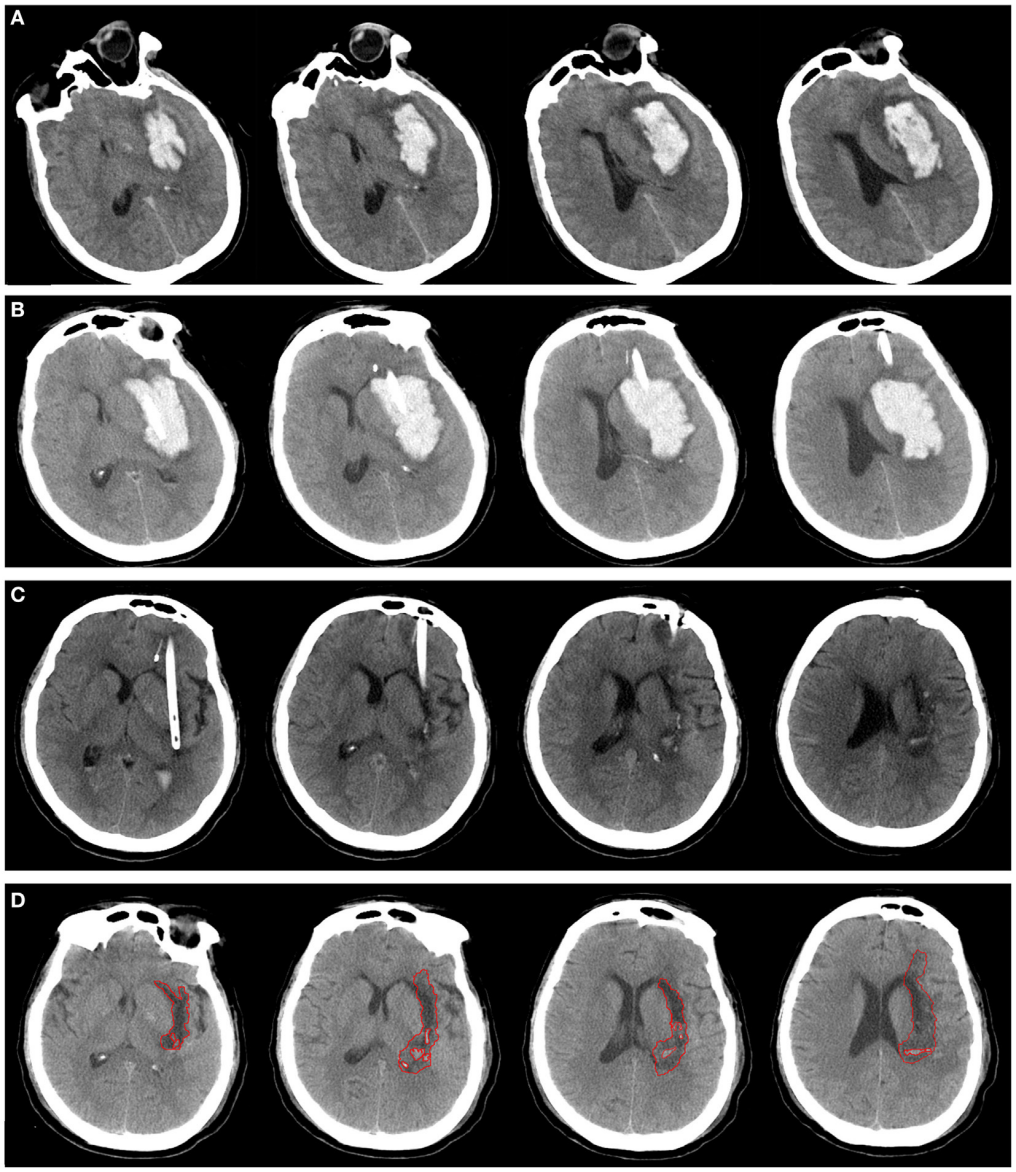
A patient with spontaneous intracerebral hemorrhage in basal ganglia received urokinase-type plasminogen activator (uPA) at 24 h postoperatively. Computed tomography scan before operation (A), at postoperative day 1 (B), after application of uPA (C), and postictus day 7 (D). The perihematomal edema was semiautomatically threshold-based segmentated and outlined with red line (D).
